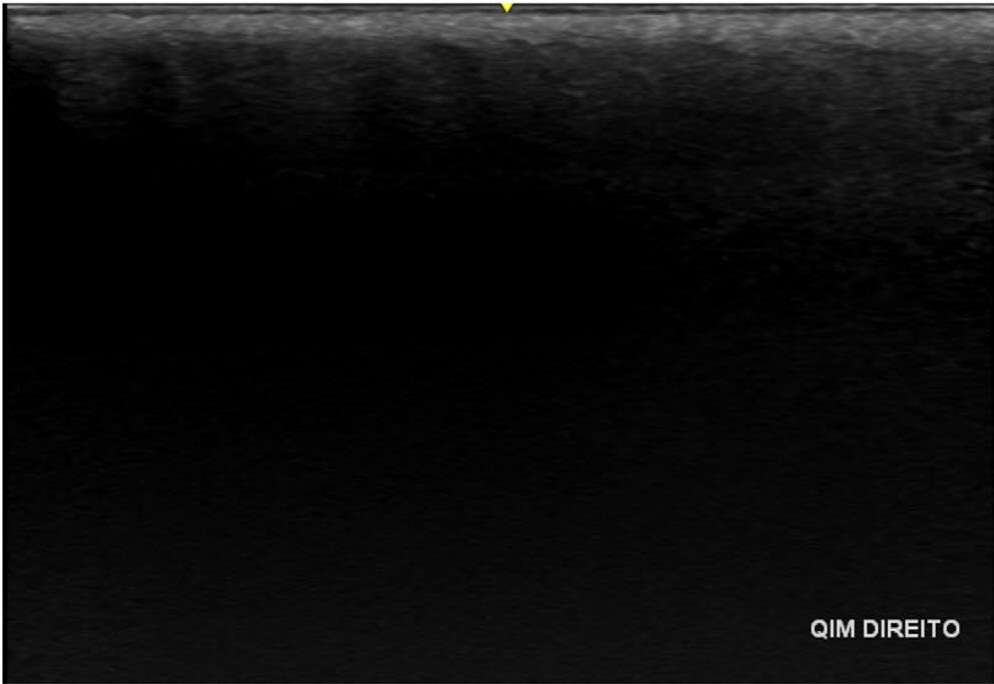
First silicone oil. Ultrasound images showing infiltrative echogenic silicone oil in the epidermis, producing a characteristic “snowstorm” artifact. Anechoic vesicles, taller than wide, are interspersed within the filler, with a posterior echogenic linear artifact indicative of pure silicone.
